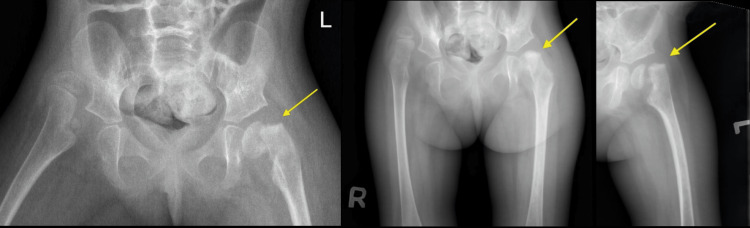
The X-ray of the patient taken six weeks post-injury
